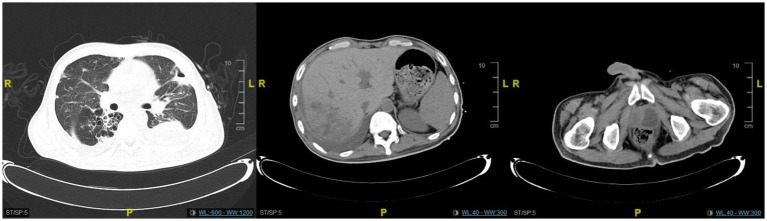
The CT scan revealed significant resolution of the lung and liver abscesses, while the prostate abscess showed only slight improvement.
